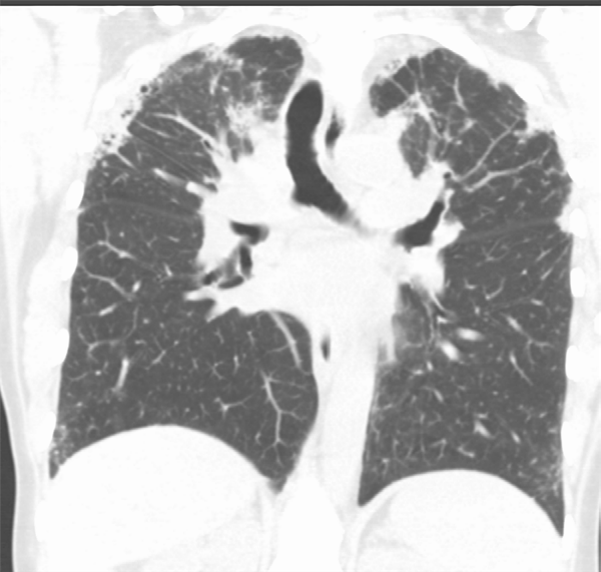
Pleuroparenchymal fibroelastosis in a 48-year-old woman. Coronal CT image shows dense subpleural consolidative abnormality with traction bronchiectasis and marked upper lobe volume loss.
